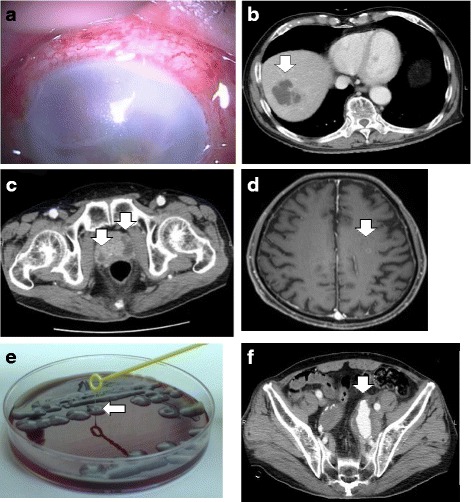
Findings from the first case. a Findings of remarkable edema and opacity in the cornea. b Contrast-enhanced whole-body computed tomography (CT) reveals a liver abscess (*white arrow*). c Contrast-enhanced whole-body CT reveals prostate abscesses (*white arrows*). d Contrast-enhanced head magnetic resonance imaging reveals a brain abscess (*white arrow*). e The first case provided colonies on the agar plate that stretched for >5 mm using the inoculation loop (*white arrows*), which indicates a positive string test result. f Contrast-enhanced whole-body CT reveals the enlargement of an existing left internal iliac artery aneurysm (*white arrow*)
